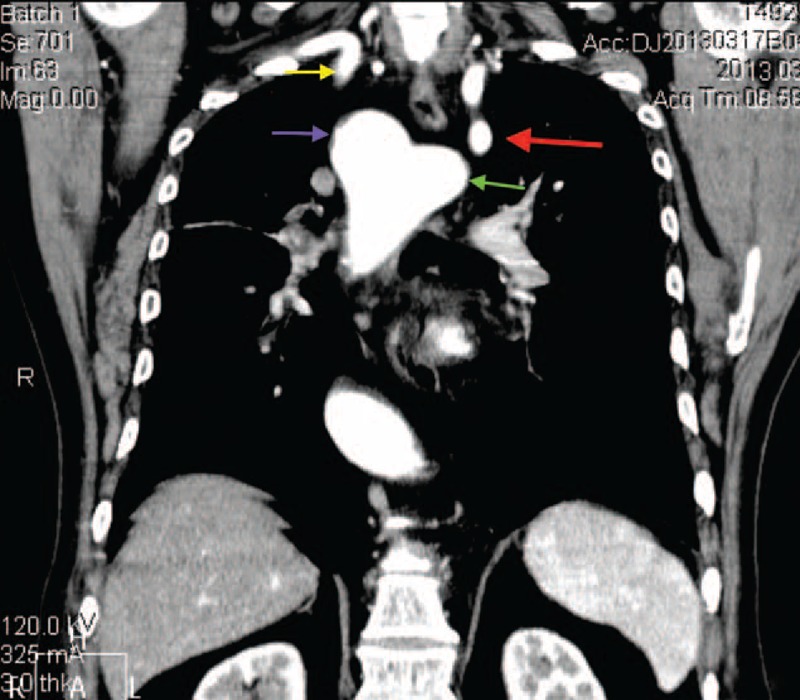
CT scan image. Anterior view. RALS. (Purple arrow, right aortic arch; red arrow, aberrant left subclavian artery; yellow arrow, right subclavian artery; green arrow, Kommerell diverticulum.) CT = computed tomography, RALS = right aortic arch with aberrant left subclavian artery.
